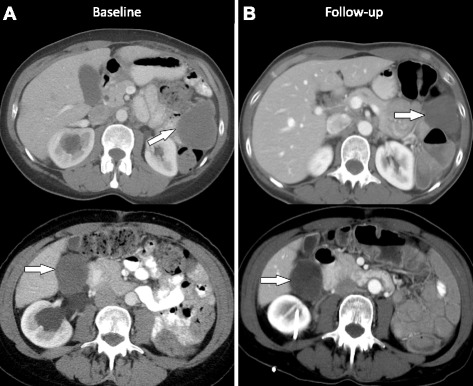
Imaging studies at baseline and follow-up. a Pre-treatment axial CT image shows a 6.2 × 5.1 cm peritoneal implant in the left upper quadrant (*top*) and a 4.4 × 4 cm peritoneal implant in the right hepatorenal space (*bottom*). b Follow-up axial CT image shows interval decrease in the peritoneal implant in the left upper quadrant which measures 4.5 × 3.8 cm, previously 6.2 × 5.1 cm (*top*). Axial CT image shows that the 4.4 × 4 cm peritoneal implant in the right hepatorenal space (*bottom*) has not changed much in size (measured 4.4 × 3.7 cm), but the lesion has now become less dense. This is an important observation as this represents response to therapy. Radiologists and clinicians should be aware that many targeted therapies may not result in significant change in size particularly during the early stages but may cause interval decrease in vascularity; on CT, this may be seen as decrease in density of the target lesions and represents response to therapy
